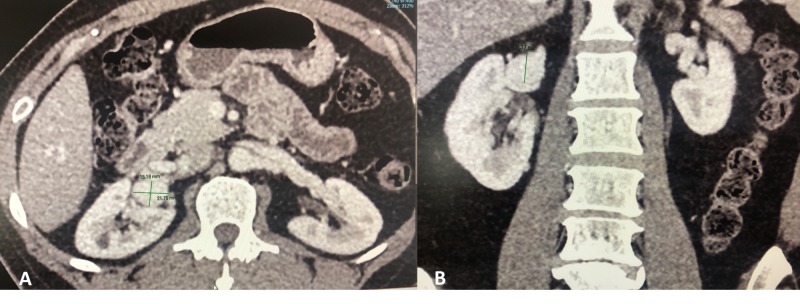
Axial (A) and coronal (B) CT images showing a right renal artery aneurysm measuring 21 x 15 x 17 mm CT: computed tomography
